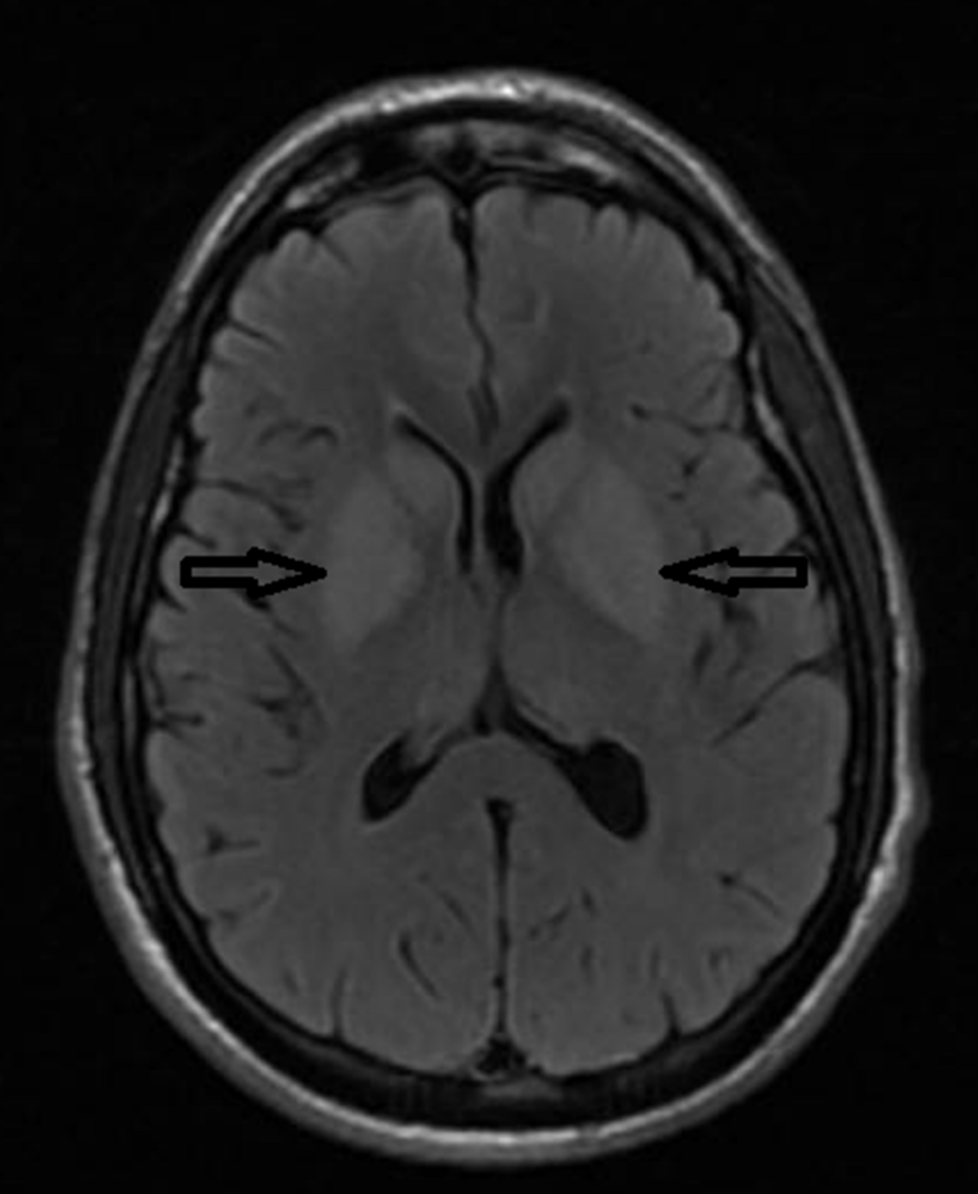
MRI brain axial T2 FLAIR sequence showing hyperintensities in bilateral basal ganglia. MRI: Magnetic resonance imaging; FLAIR: Fluid attenuated inversion recovery.
